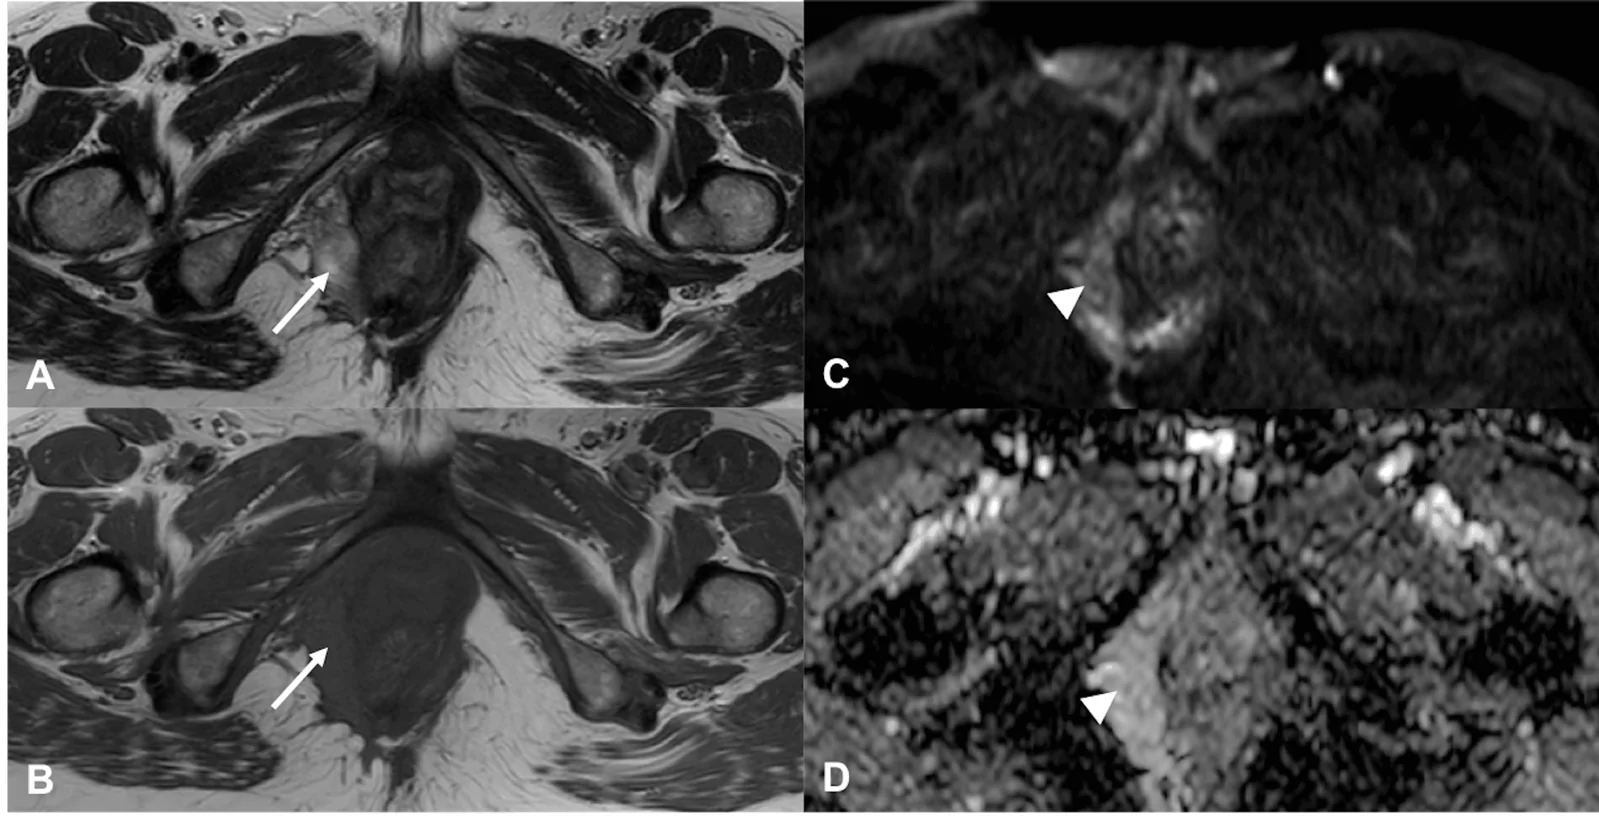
Recurrent tumor at one-year MRI follow-up A, B) Axial T2-weighted (A) and T1-weighted (B) images demonstrate recurrent disease in the right ischiorectal fossa with signal characteristics similar to those of the primary tumor (arrows), measuring 35 × 20 mm (transverse × anteroposterior). C, D) No diffusion restriction is demonstrated on diffusion-weighted imaging (DWI) (C), with a corresponding high apparent diffusion coefficient (ADC) value of 1.78 × 10⁻³ mm²/s on the ADC map (D) (arrowheads).
